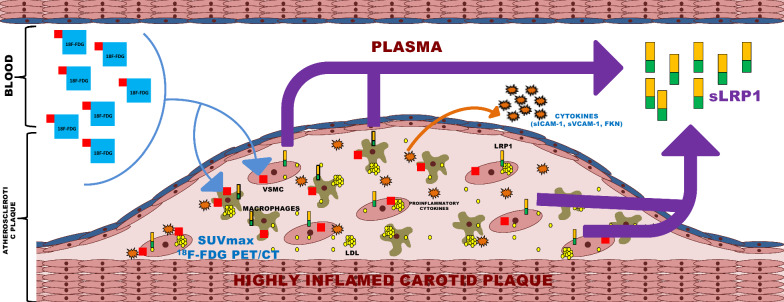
The progression of atherosclerotic plaque is associated with an increased number of active cells in the arterial intima (macrophages and smooth muscle cells) that avidly take up ^18^F-FDG (SUVmax). Many of these cells become foam cells by taking up atherogenic lipoproteins retained in the intimal extracellular matrix, acquiring a highly proinflammatory phenotype that release cytokine and sLRP1 to the circulation. The results of the present study show the close relationship between sLRP1 and proinflammatory cytokines (sICAM-1, sVCAM-1 and FKN) in the blood, as well as between circulating sLRP1 levels and intra-plaque inflammation measured by PET in patients with a recent ischemic stroke. ^*18*^*F-FDG* 18F-fluorodeoxyglucose, *SUVmax* standardized uptake value, *sLRP1* soluble low-density lipoprotein receptor-related protein 1, *sICAM-1* soluble intercellular cell adhesion molecule 1, *sVCAM-1* soluble vascular adhesion molecule 1 (sVCAM-1), *FKN* fractalkine
